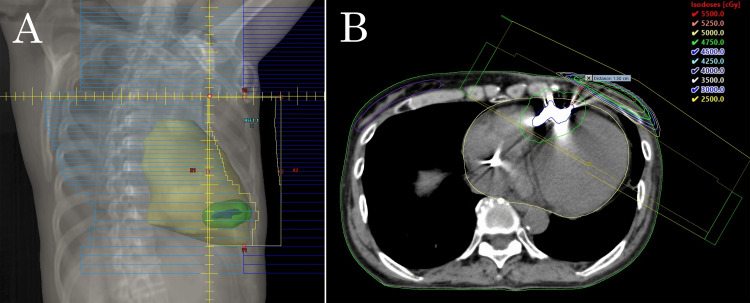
Dose distribution from the initial 3D conformal plan. The heart (yellow), leadless pacemaker (LPM) (blue), planning organ-at-risk volume (PRV_LPM) (green), and right breast (purple) are contoured. (A) Digitally reconstructed radiograph of the chest wall field with a gantry angle of 300°. (B) Multileaf collimators were configured to maintain a 1.5-cm distance from the LPM for shielding, and a 1-cm isotropic margin was applied to define the PRV_LPM. Nevertheless, the LPM received a maximum dose of 314 cGy and the PRV_LPM 565 cGy, both exceeding institutional dose constraints.
